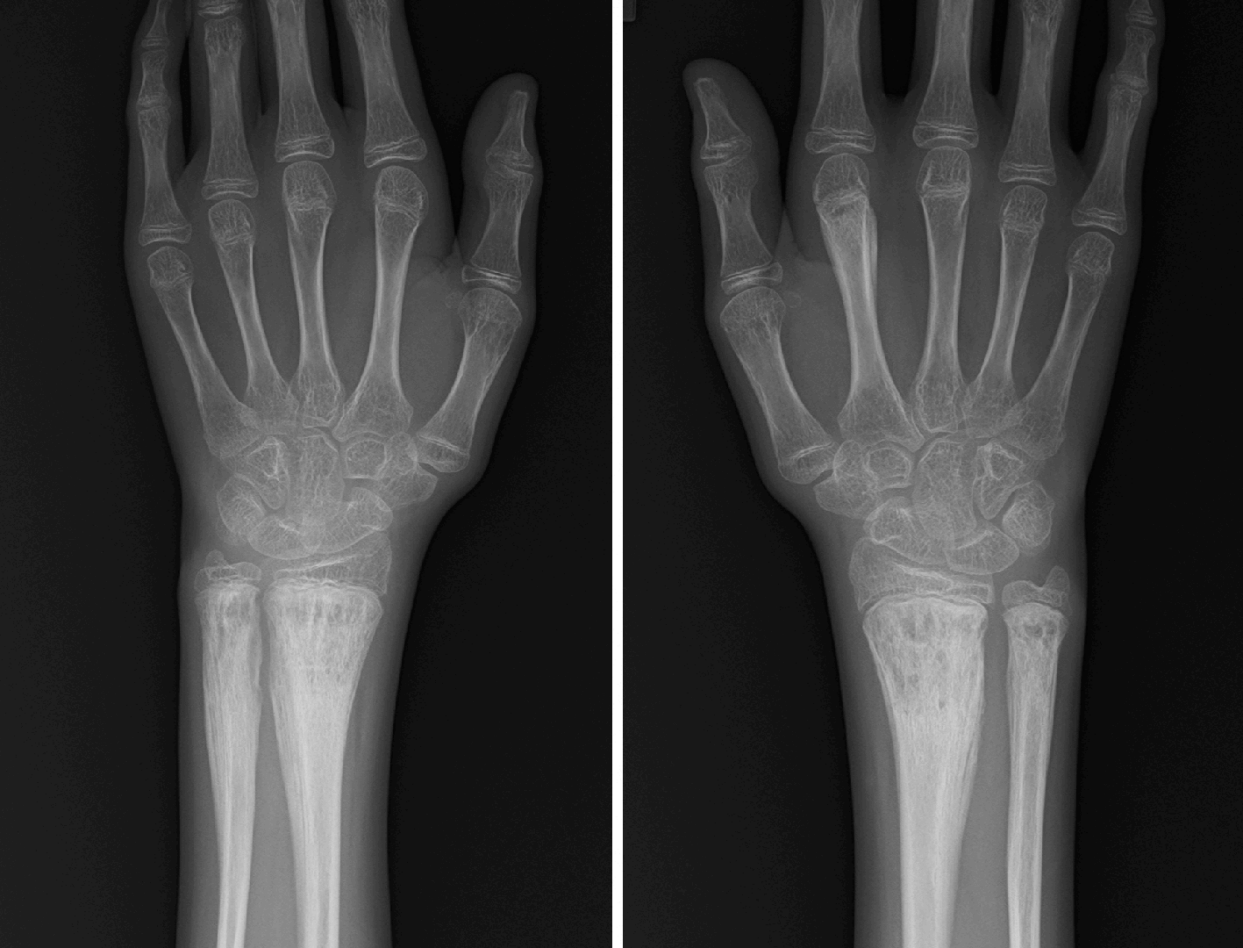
Radiograph of both wrists performed 6 months after the initial visit. Radiographs showing chronic osteomyelitis, though the contralateral wrist was asymptomatic.
